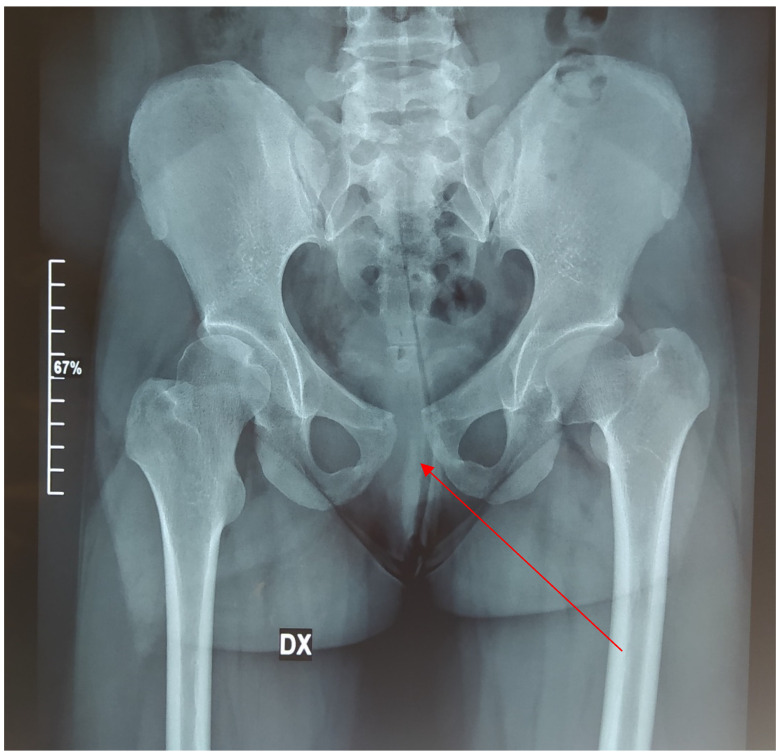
Pelvis radiography in 2021 with a wide pubic symphysis (red arrow).
